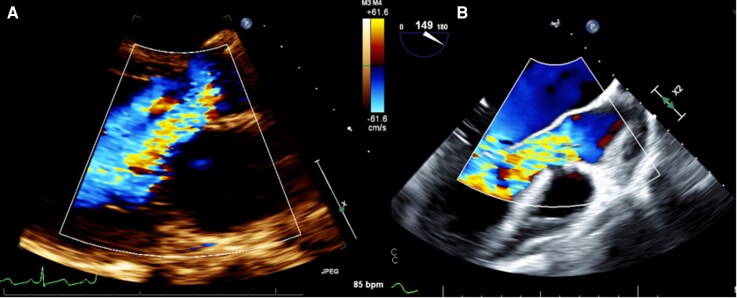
Echocardiographic demonstration of progressive aortic regurgitation. (*A*) Follow-up transthoracic echocardiography (parasternal long-axis view) demonstrating worsening aortic regurgitation. Pulsed-wave Doppler of the abdominal aorta shows prominent holodiastolic flow reversal, consistent with severe aortic regurgitation. (*B*) Transoesophageal echocardiography (mid-oesophageal long-axis view) demonstrating severe aortic regurgitation on colour Doppler.
